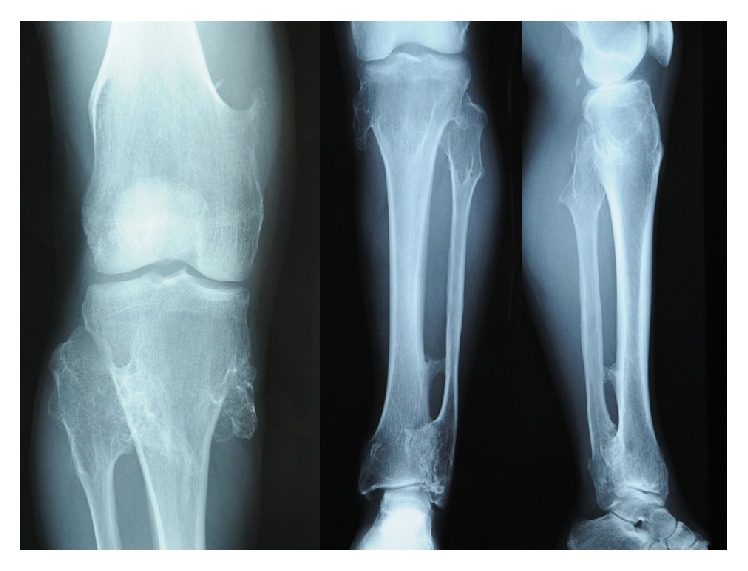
Radiographs of the right knee joint showing osseous projection.
